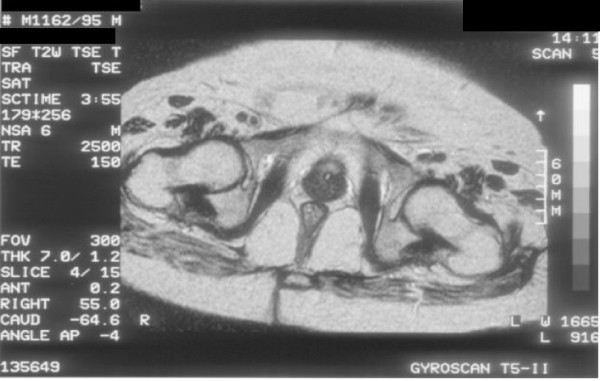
Magnetic Resonance Imaging of pelvis performed on 23 October 1995: Axial T-2 weighted image shows no extra-capsular spread of carcinoma of prostate.
